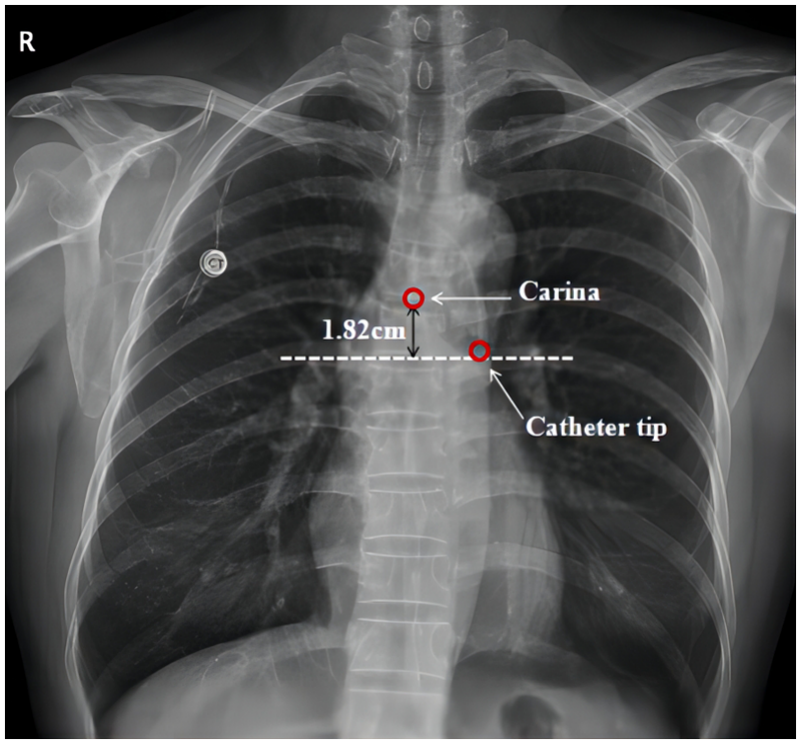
Postoperative chest x-ray showed the catheter tip is located 1.82 cm below tracheal carina and considered the ideal location.
